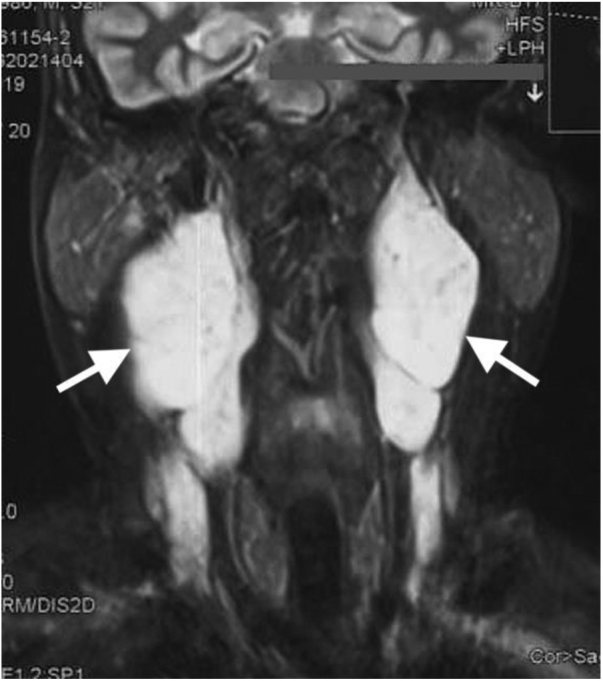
Coronal T2-weighted MRI image shows bilateral hyperintense masses (white arrows) at parapharyngeal space with heterogeneous contrast enhancement.
